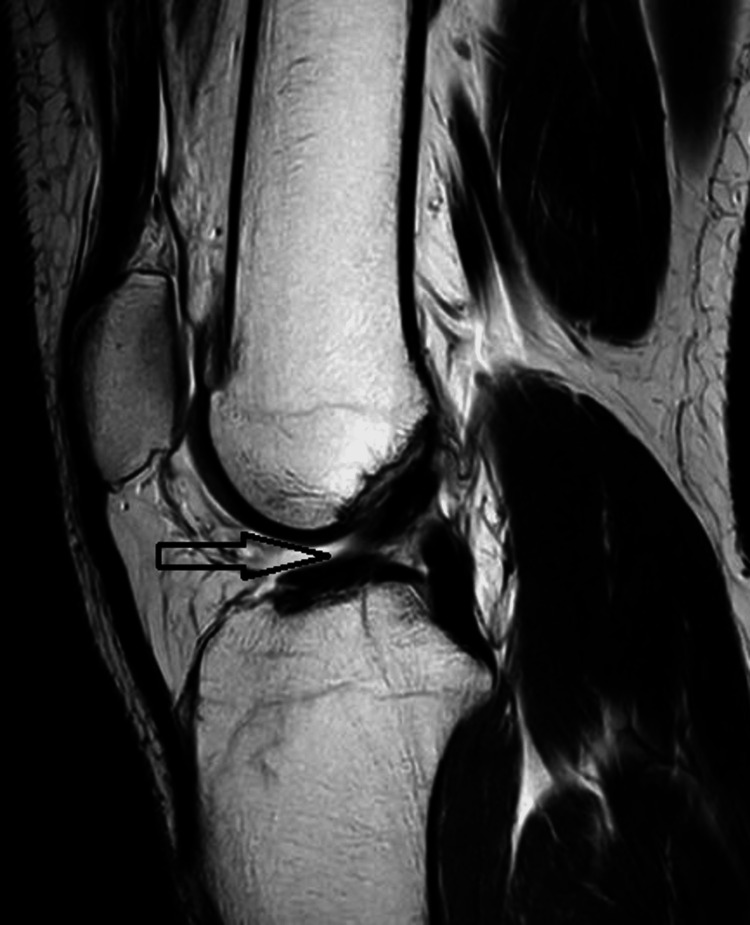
Right knee MRI image The image shows a complete ACL tear in the right knee.
